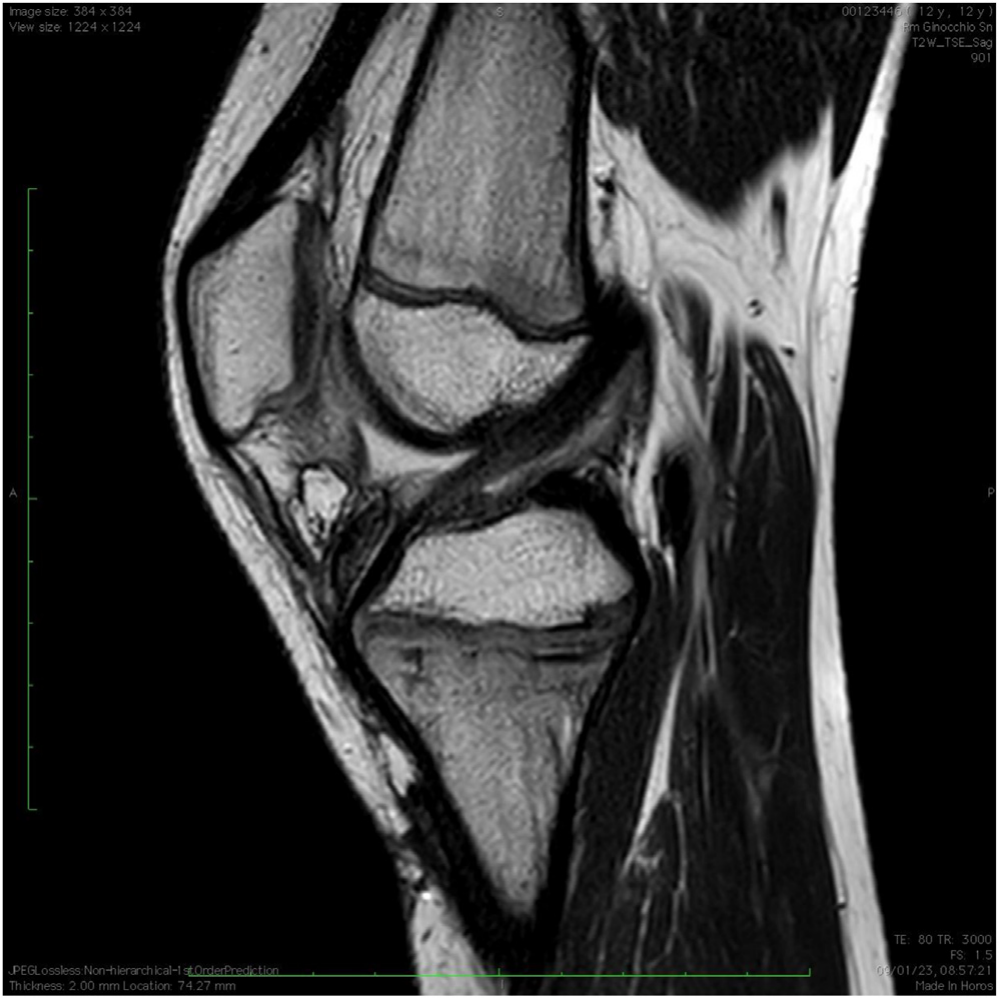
A sagittal, STIR (short-tau-inversion-recovery) sequence magnetic resonance imaging scan of a combined intra-articular anterior cruciate ligament (ACL) reconstruction and lateral extraarticular tenodesis with the described technique. This sequence shows the spearing of the femoral and tibial growth plates and good signal intensity and morphology of the ACL graft at 6 months after reconstruction.
